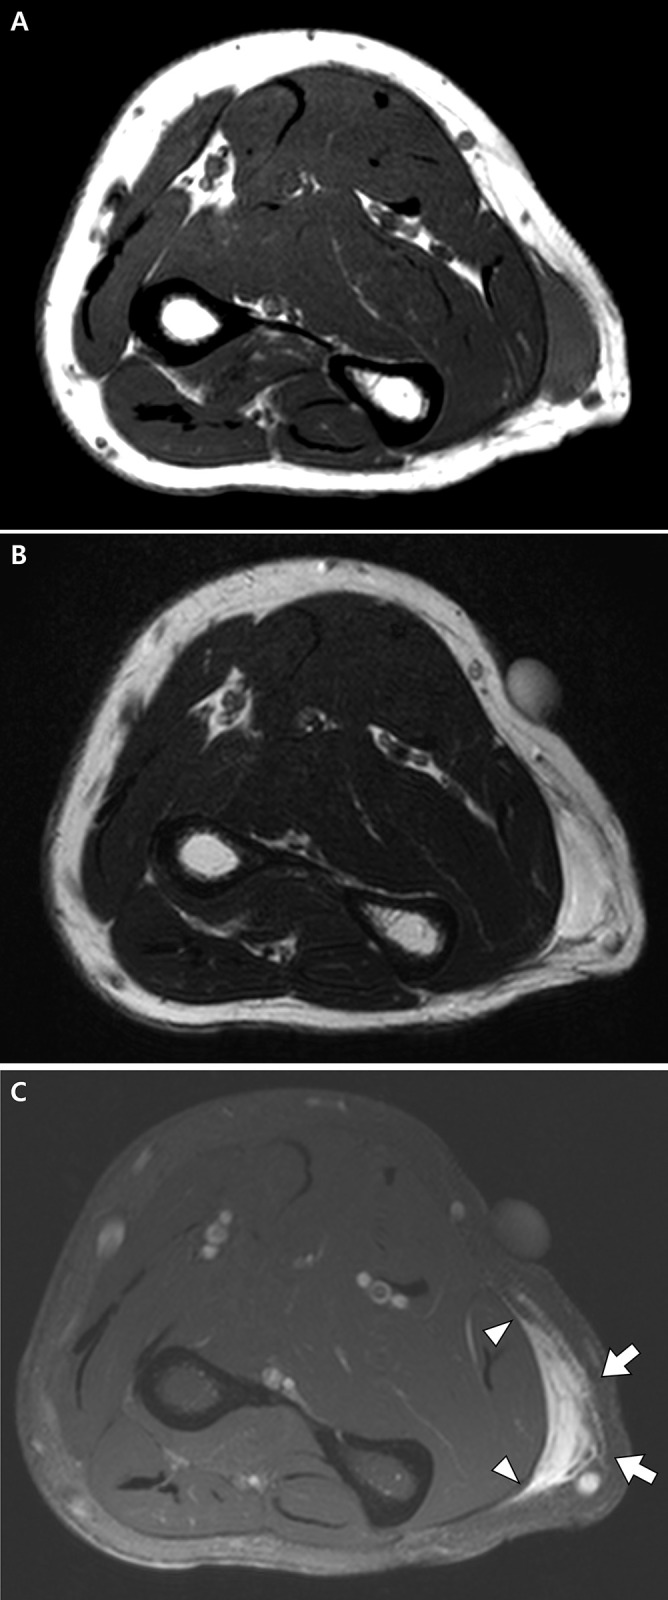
Magnetic resonance images of a 66-year-old man with myxofibrosarcoma before unplanned excision. A Axial T1- and B T2-weighted images of the right forearm show a 2.7 cm-sized mass in the subcutaneous fat layer. C Peritumoral abnormality is noted as an infiltrative enhancement along the superficial (arrows) and deep peripheral fascia (arrowheads) on axial fat-suppressed contrast-enhanced T1-weighted image.
